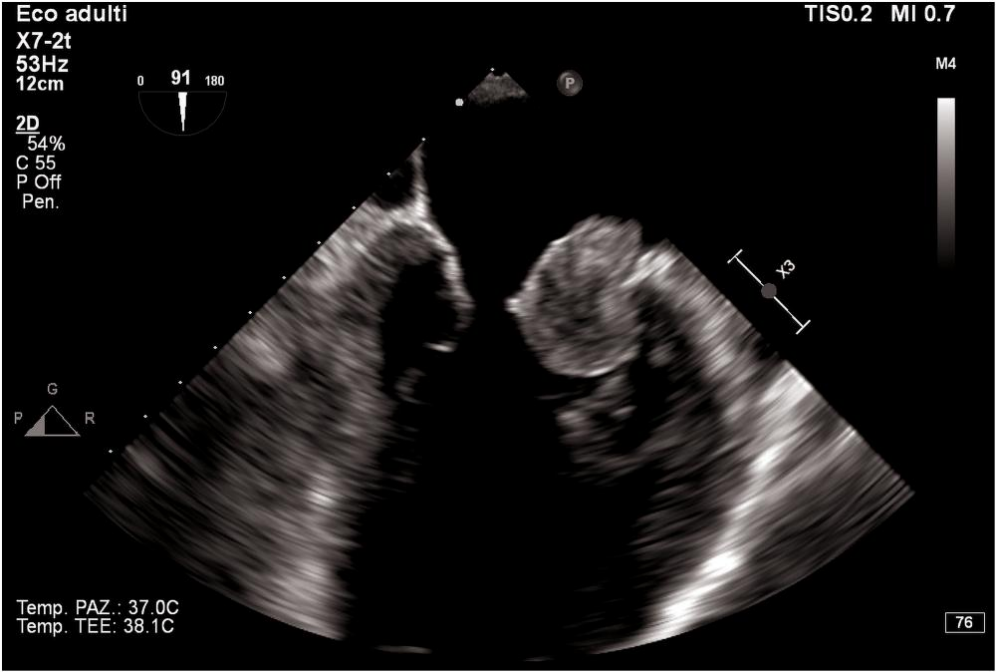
Transoesophageal echocardiogram mid-oesophageal view at 90° (two-chamber view). The figure shows the mass protruding in the left ventricle.
